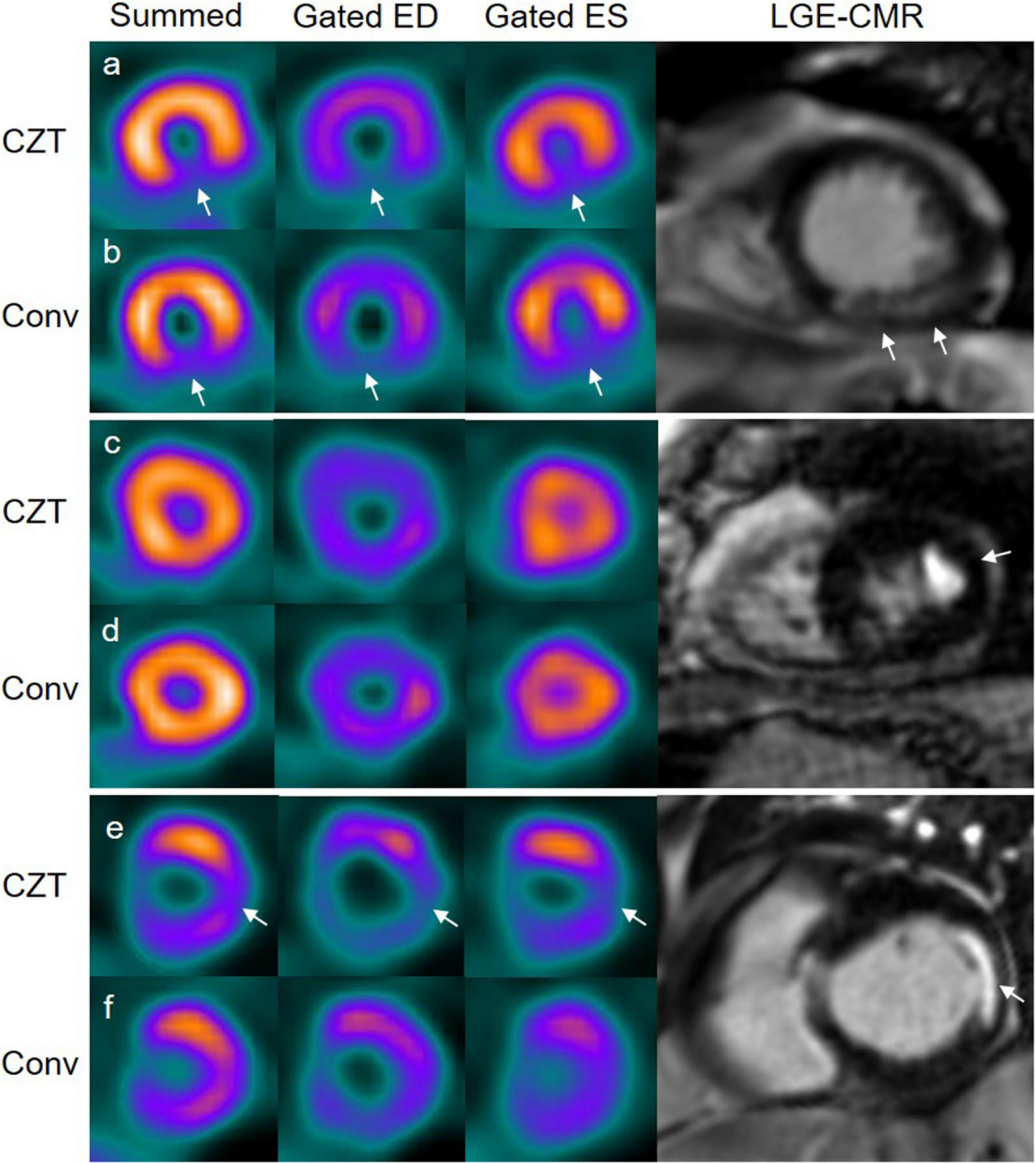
Patient examples of myocardial infarct (MI) by cardiac magnetic resonance (CMR), myocardial perfusion SPECT (MPS) with a cadmium-zinc-telluride (CZT) gamma camera and a conventional (Conv) gamma camera. Columns from left to right show summed MPS perfusion images, gated MPS in end-diastole (ED) and end-systole (ES) and late gadolinium enhancement (LGE)-CMR. Case *a* and *b* are examples of an MI in the apical inferior wall on CMR which is correctly diagnosed by both CZT and conventional MPS (arrows). Case *c* and *d* are examples of an MI in the apical lateral wall on CMR (arrow), missed by both CZT and conventional MPS. Case *e* and *f* are examples of an MI in the basal lateral wall on CMR correctly diagnosed by CZT MPS (arrows) but missed by conventional MPS
